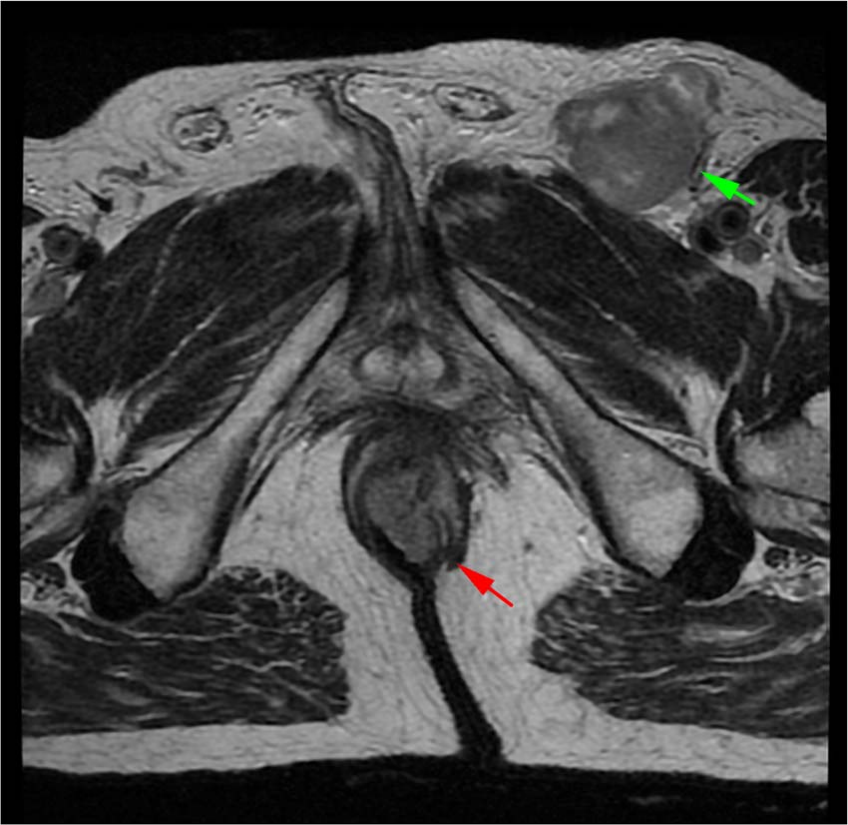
MRI of Case 2 shows an infiltrating anal tumor involving the internal and external sphincters, as well as perianal adipose tissue (lower arrow), with associated inguinal lymphadenopathy (upper arrow). The tumor demonstrates high signal intensity on axial T2-weighted imaging.
